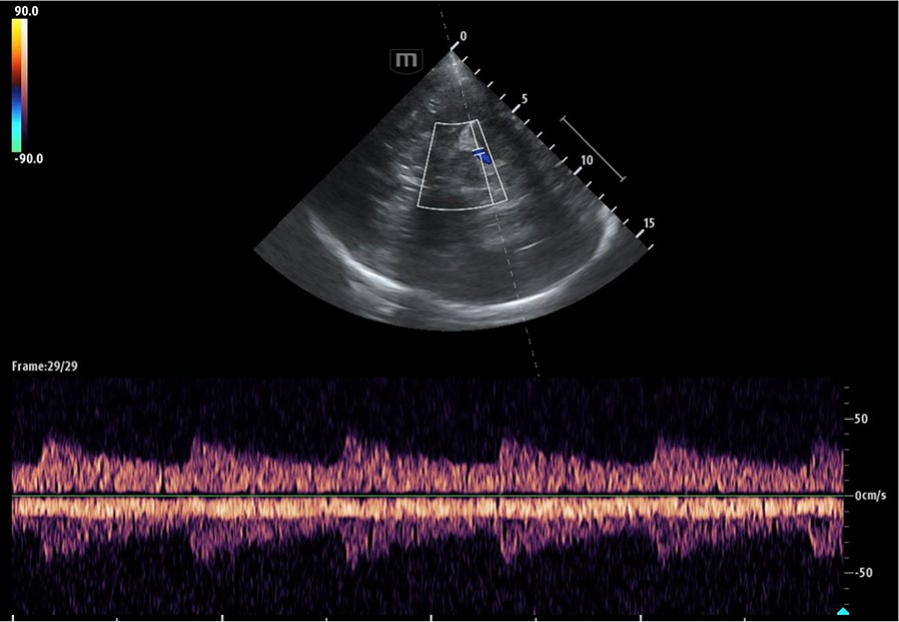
Transcranial colour-coded triplex and pulse-wave Doppler of the branching posterior cerebral artery with additional basal vein of Rosenthal waveform demonstrated within spectral Doppler trace
